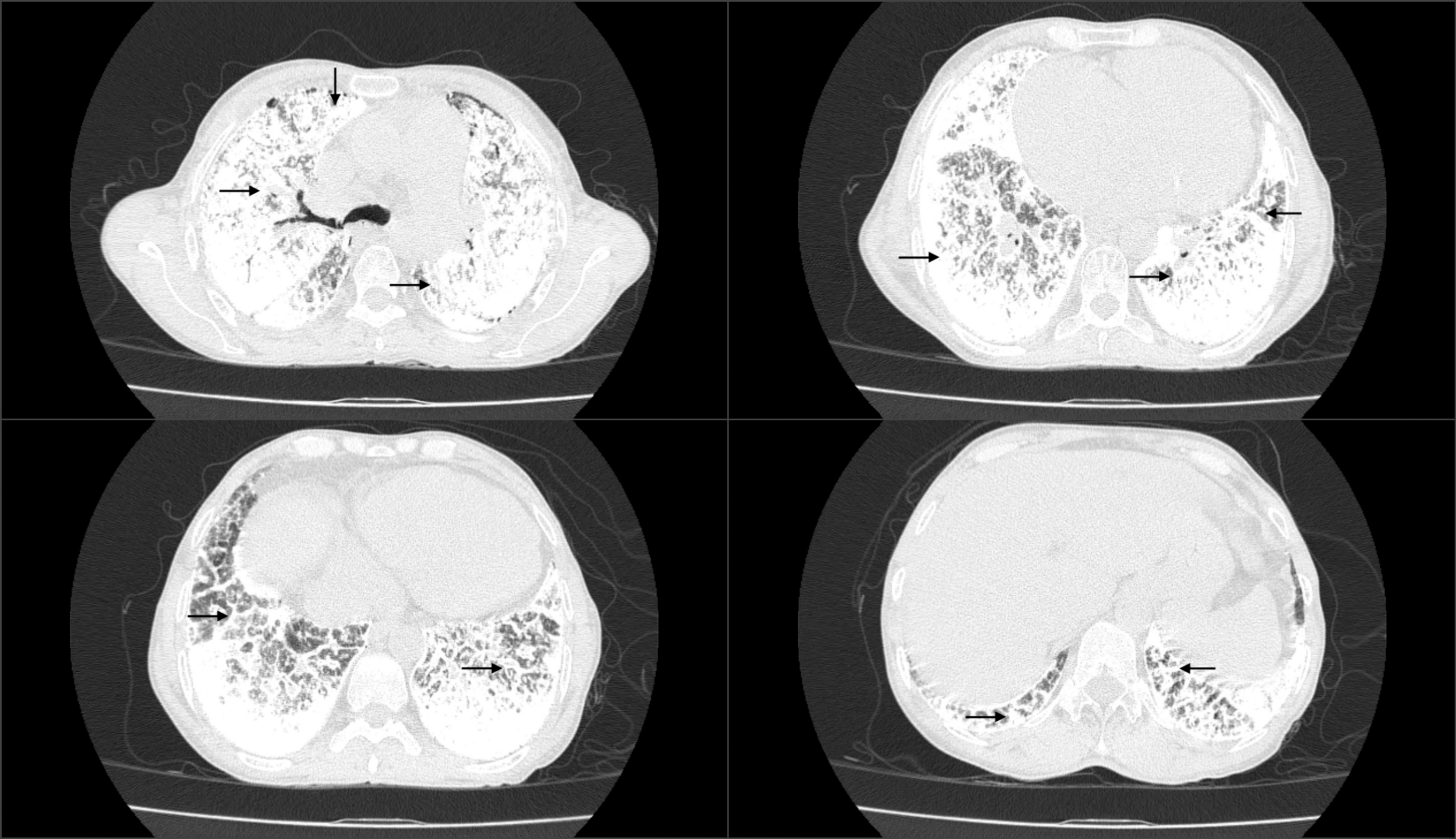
Hight-resolution CT of the chest demonstrating extensive micro-nodular and macro-nodular calcifications - "sandstorm" appearance in both lungs.
